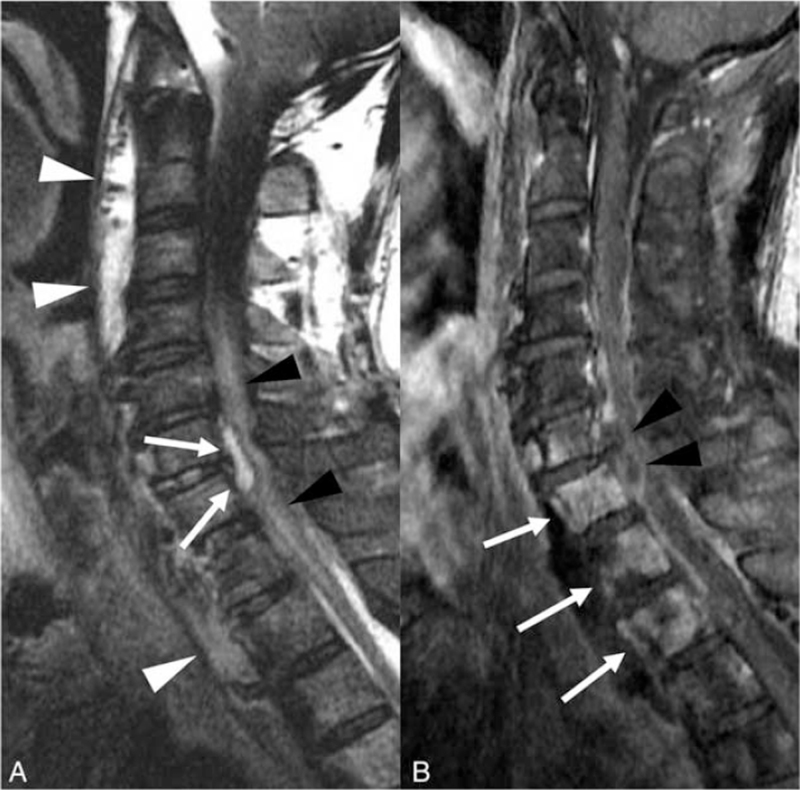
(A) The sagittal fat-saturated T2-weighted C-spine magnetic resonance imaging (MRI) shows anterior epidural fluid (arrow) in C6–7 displaces the thecal sac posteriorly. The spinal cord exhibits a diffuse, high signal intensity (black arrows heads), attributable to compression by the abscess. Also, a diffuse prevertebral abscess from C2 to T3 is apparent on the T2-weighted image (white arrowheads). (B) The sagittal T1-weighted enhanced C-spine MRI shows rim enhancement on epidural fluid collection (black arrowheads), suggesting an epidural abscess. The vertebral bodies (C6–T2) exhibit high signal intensity on the T2-weighted image and enhancement (arrows) on the T1 weighted-image, compatible with pyogenic spondylitis.
